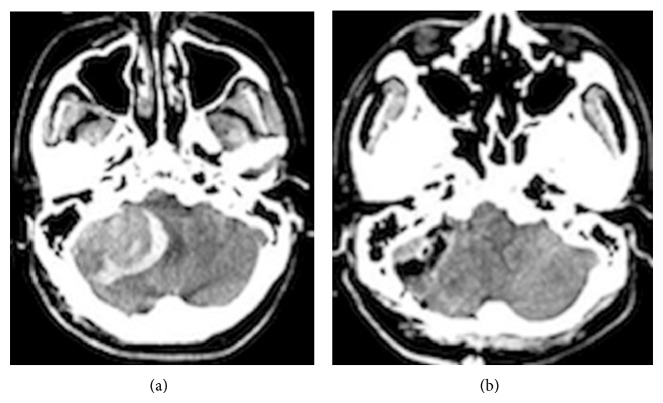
Computed tomography (CT) revealing infratentorial tumor with massive hematoma around the tumor, compressing the brainstem (a). Postoperative CT shows complete removal of the tumor and hematoma (b).
